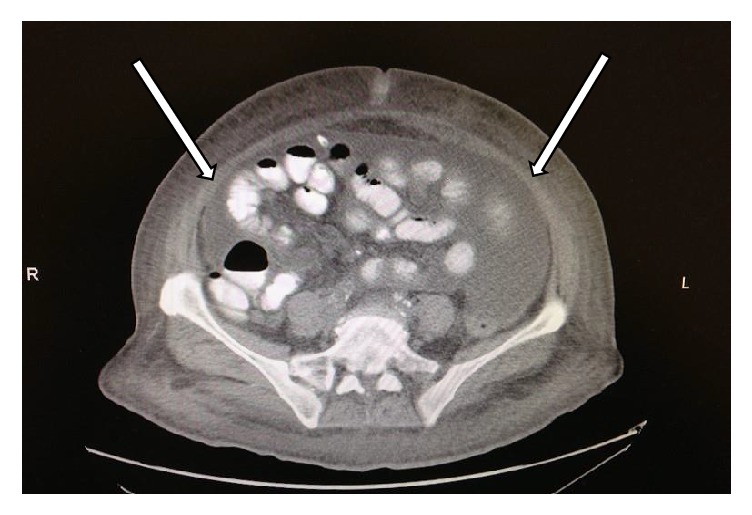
Computed tomography of the abdomen showing peritoneal thickening (arrows), consistent with peritonitis.
